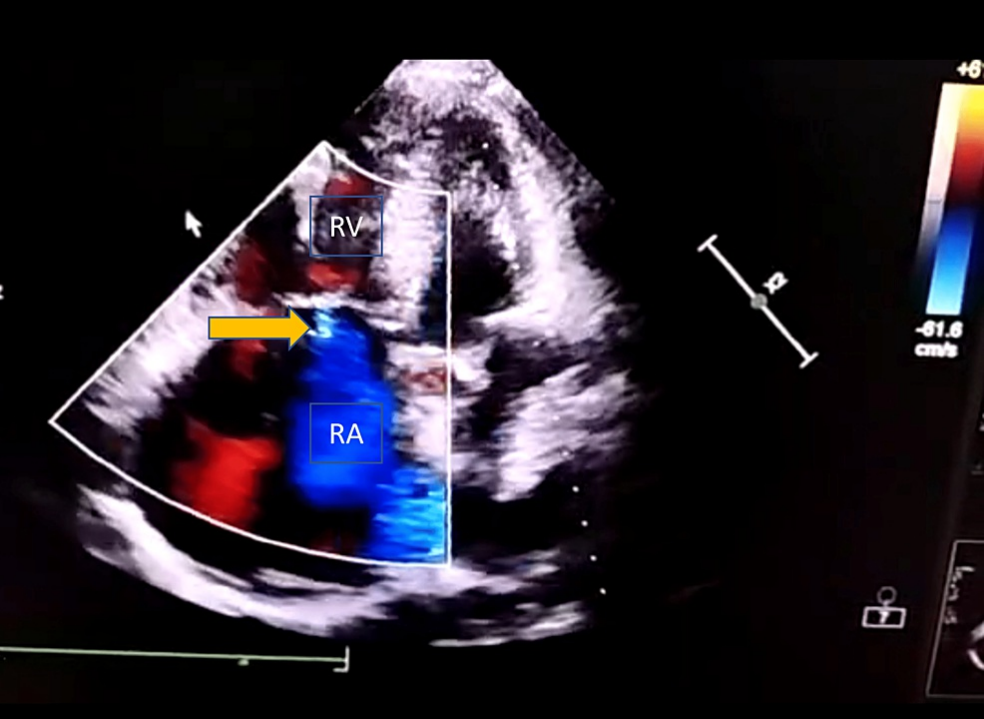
Echocardiography, four chamber view showing dilated right atrium (RA), right ventricle (RV), and tricuspid regurgitation (TR) jet (yellow arrow)
